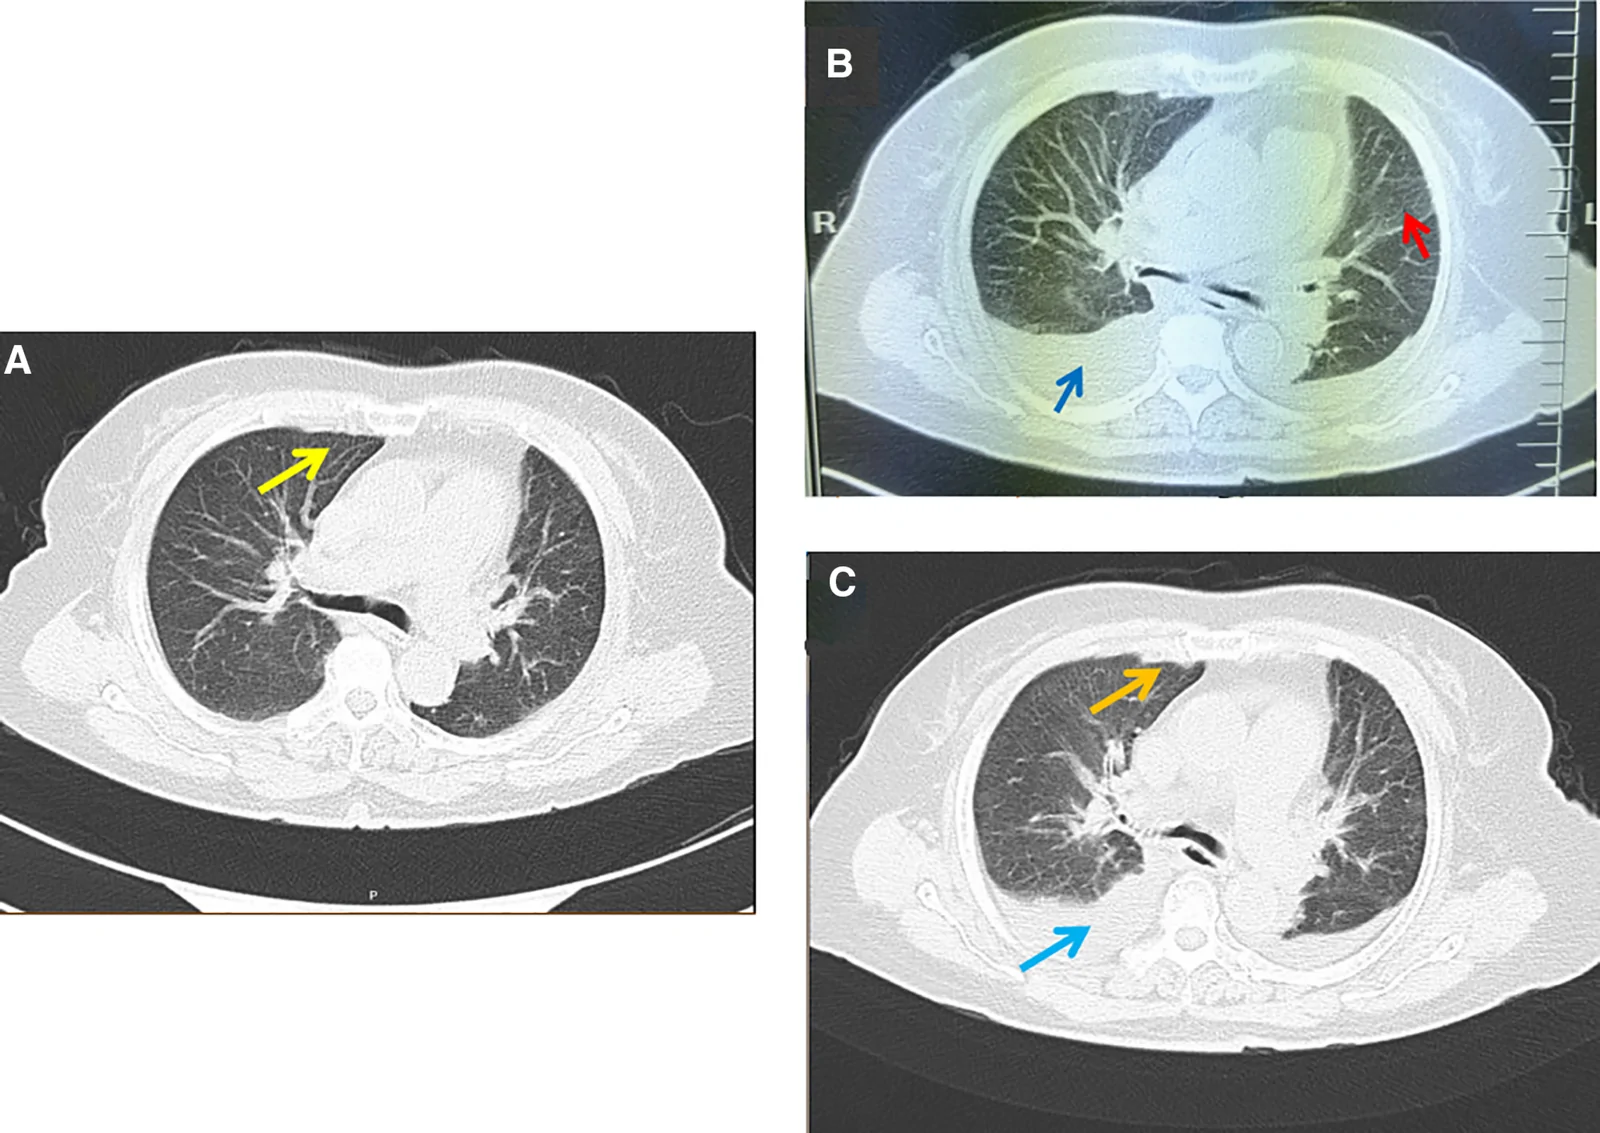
Lung CT and clinical course. (A) Chest CT obtained prior to admission, showing mild inflammatory lesions in the lungs (as indicated by the yellow arrow). (B) and (C) Chest CT performed on the third day of hospitalization, revealing multifocal inflammatory lesions in both lungs (left-sided lung infections are marked with red arrows, and right-sided lung infections are marked with orange arrows) and pleural effusion (as indicated by the blue arrow). CT = computed tomography.
